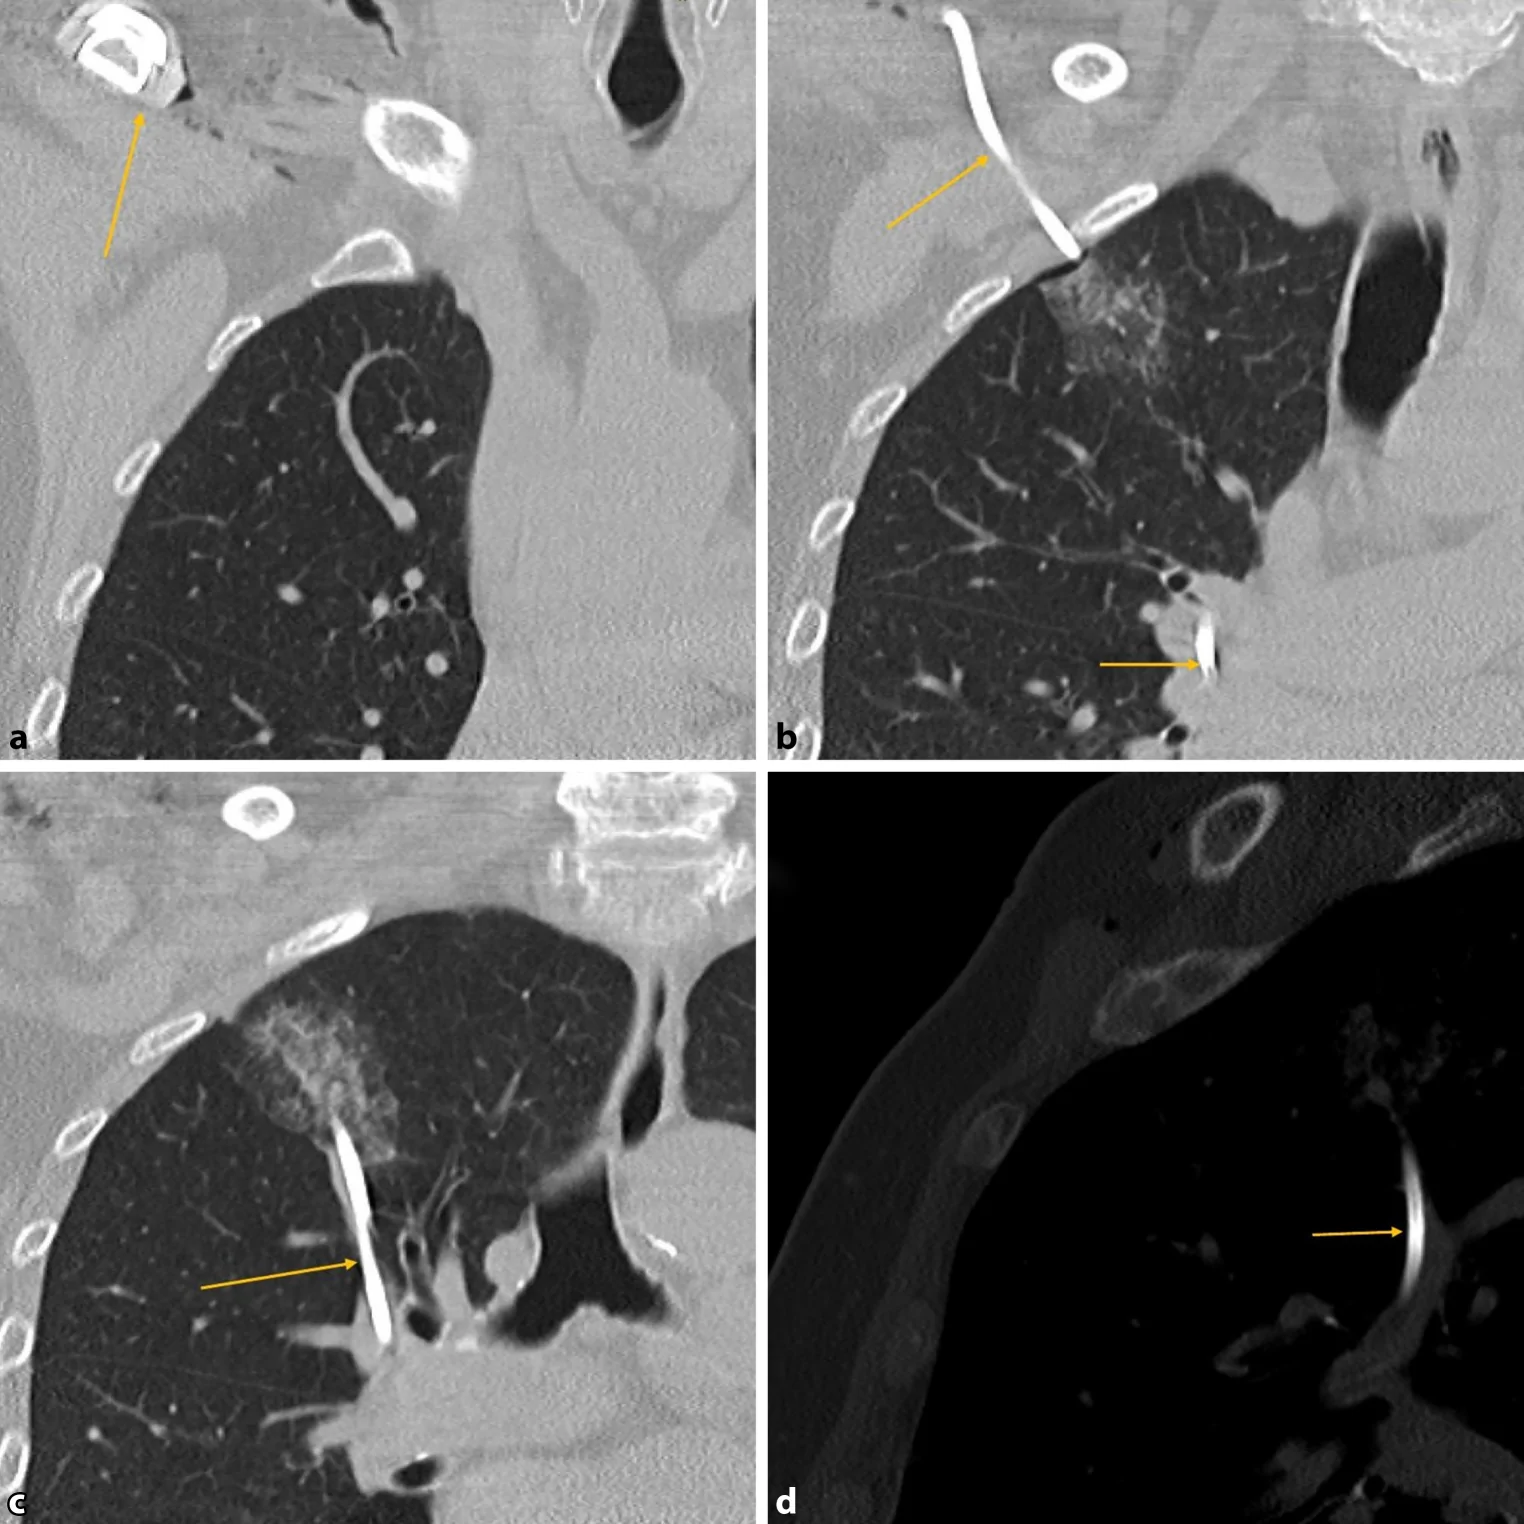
The CT images (a–d) clearly confirmed the intrapulmonary location of the port system, with the catheter positioned intravascularly in an apical segmental artery of the right upper lobe
